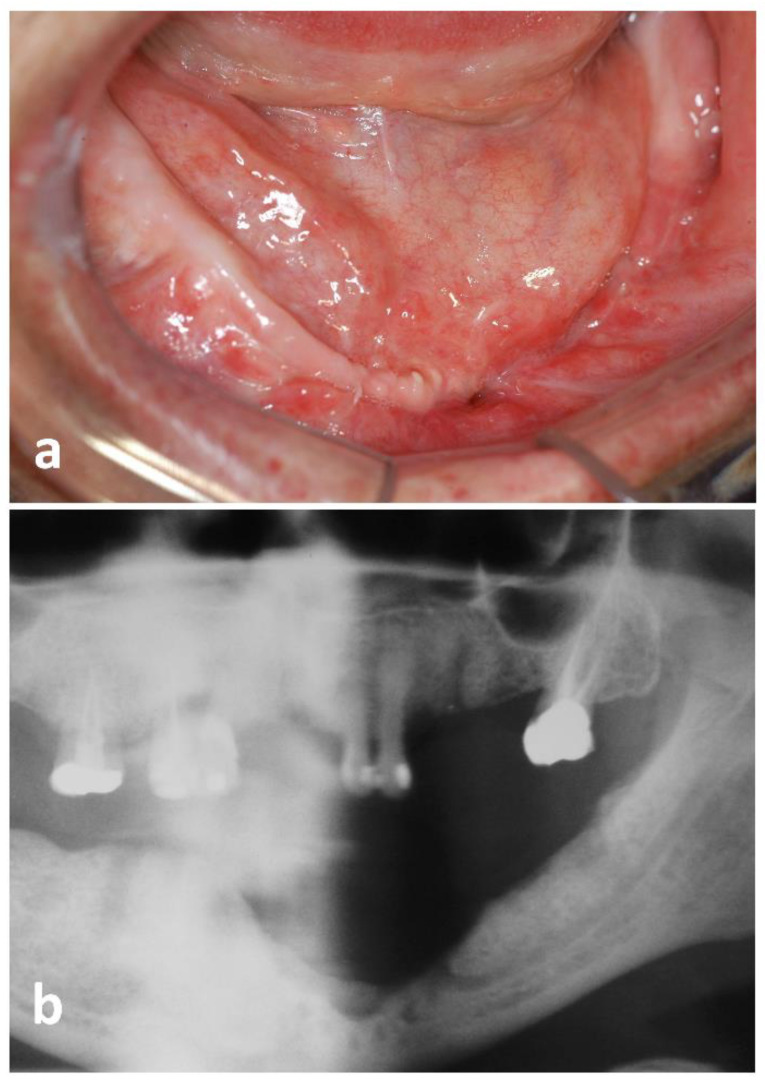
Clinical features of mandibular *Aspergillus* osteomyelitis. (a) Oral fistula and diffuse swelling on the lower left alveolar ridge. (b) Radiographic aspect, showing marginal bone resorption with poorly defined borders caused by *Aspergillus* infection in the mandible.
